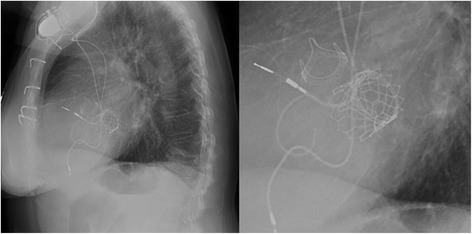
30-days Trans-Thoracic echocardiography Mitral prosthesis’ gradient
